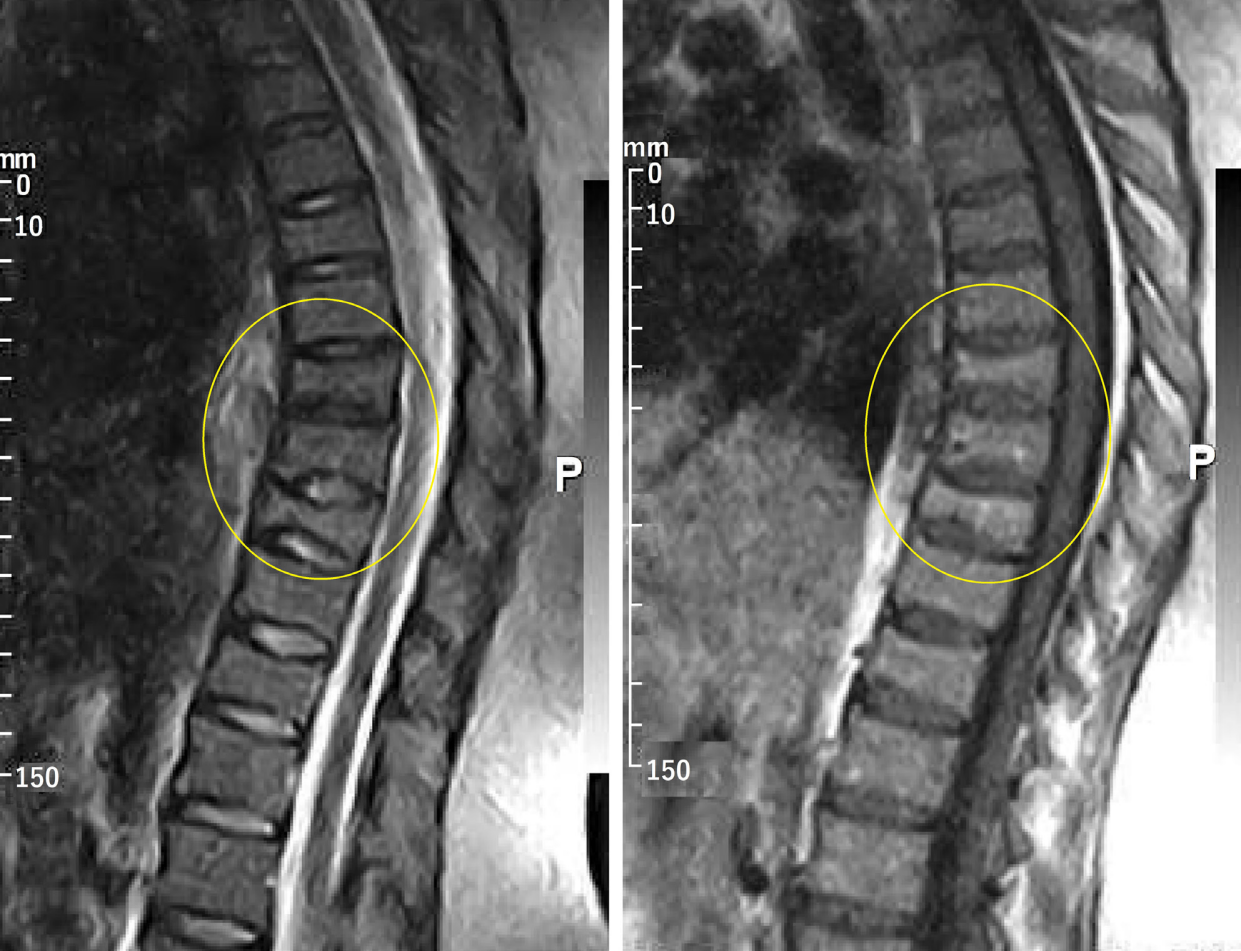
MRI of the thoracic vertebrae. Collapse is seen in T9‐T12 vertebrae.
